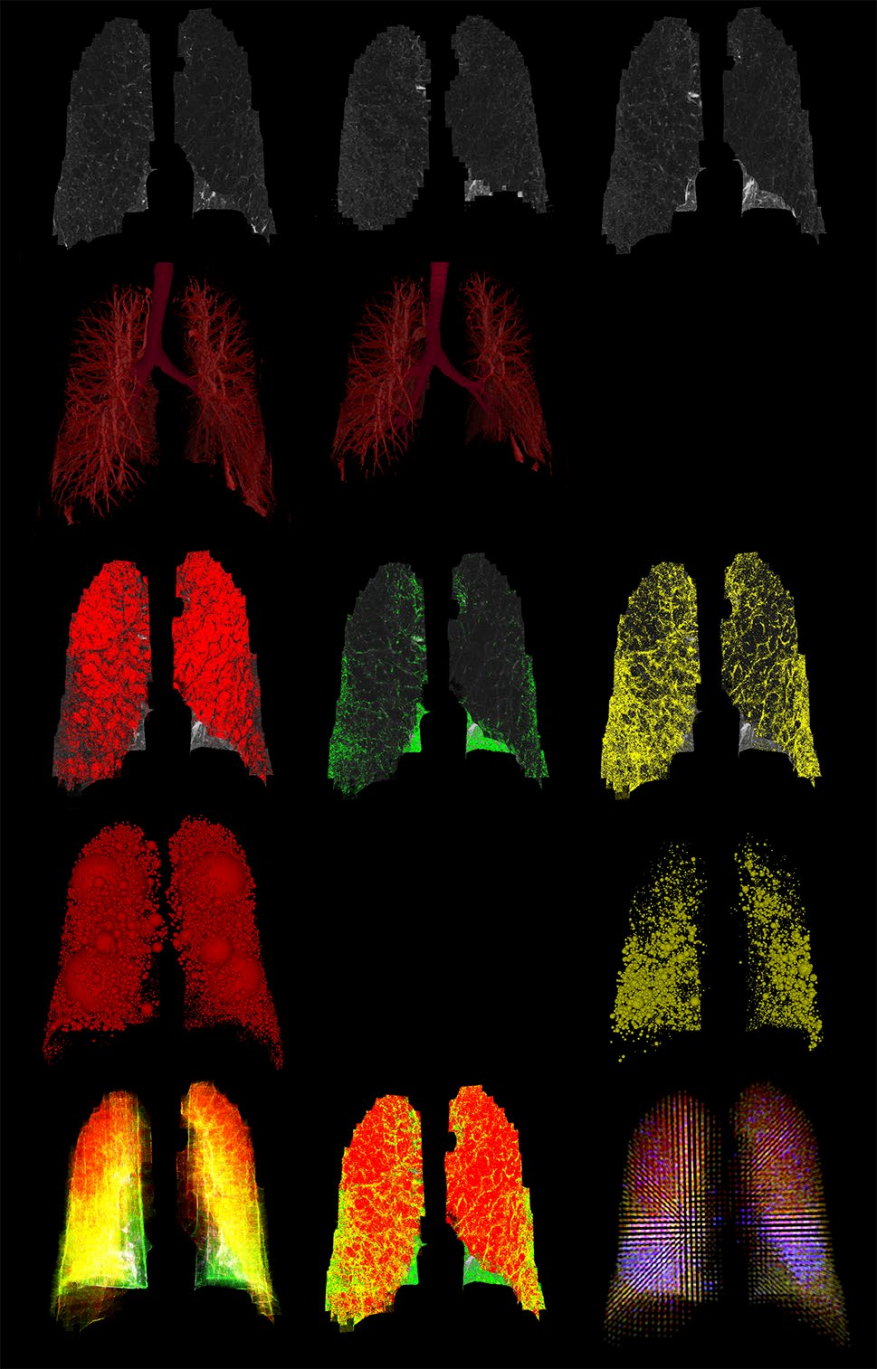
First row: left is inspiratory CT, the center is the original expiratory CT, right is the co-registered result of expiratory CT. Second row: left is the vascular tree rendering of inspiratory CT, and center is the expiratory CT. The visual inspection shows extensive pruning in lower left lobes and relatively mild pruning in lower right lobes. Third row: Red color marks the emphysema regions (HU-950), green color marks the normal regions, yellow marks the air-trapping of expiratory CT, distinguished from emphysema of inspiratory CT using technique of parametric response map (PRM). The fourth row is the result of second-row using low attenuation clusters. Fifth row: left is the visualization result of PRM in a three-dimensional model, the center is the mapping of the PRM on HU values of inspiratory CT, right is the result of regional functional prediction mark on the grids, which has blue color as the most severe candidates for the target lobes
